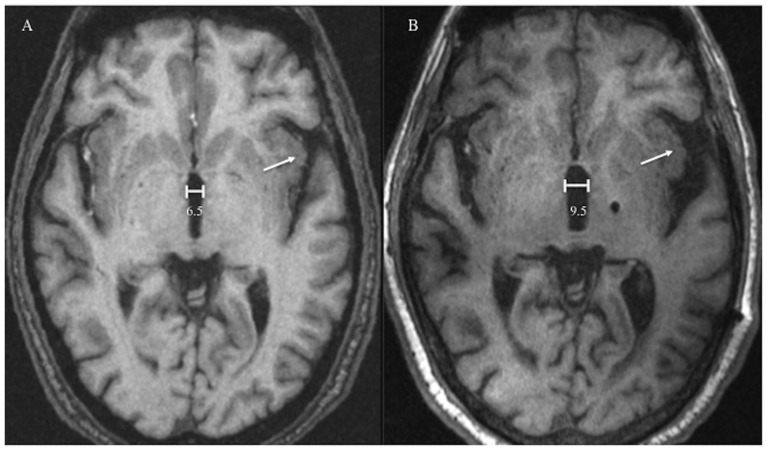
Comparison of two MRI scans over 10-year period. The post-operative scan 10 years after DBS surgery shows an increase in size of the ventricles (millimeters) and widening of the Sylvian fissure (arrow). A: Preoperative scan for DBS targeting; B: Most recent scan.
